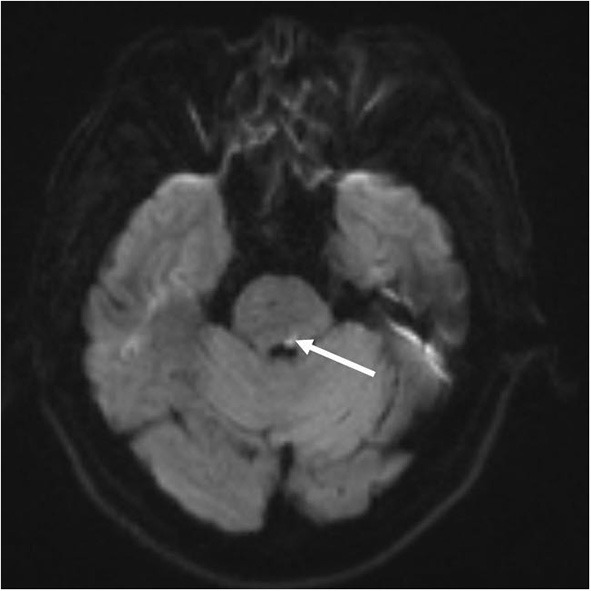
Brain MRI: Diffusion-weighted images reveal a focal ischemic stroke in the dorsal pons (arrows).
